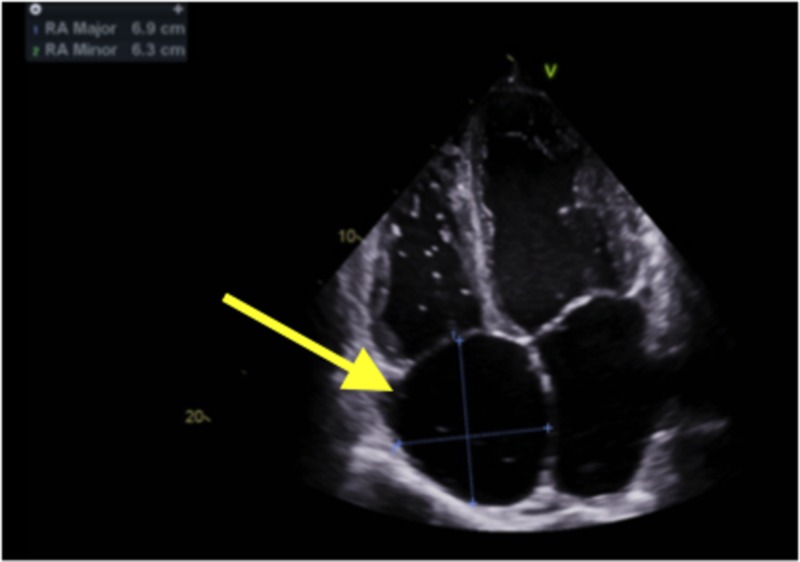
Echocardiogram: Arrow pointing toward dilated right atrium
